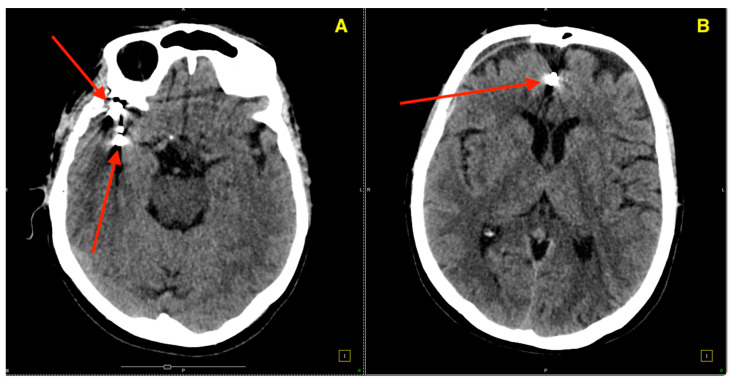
Three-month postoperative non-contrast CT scan. (A) Postoperative CT showing the aneurysm clips at the middle cerebral artery bifurcation (upper arrow) and the distal M2 segment (lower arrow), demonstrating proper placement without evidence of migration or residual filling. (B) Postoperative CT highlighting the aneurysm clip at the pericallosal artery, showing accurate positioning with no surrounding ischemic changes.
